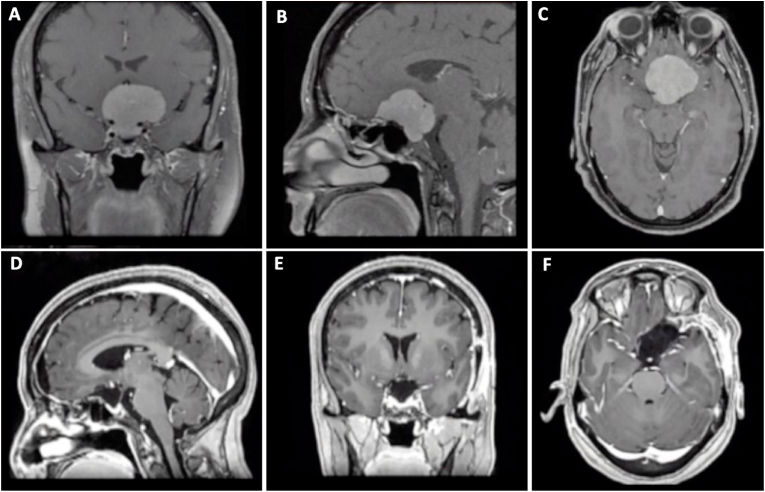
(A–C) Preoperative MRI: from left to right: coronal, sagittal, and axial views from a T1WI C+ showed an extra-axial lesion on the tuberculum sellae region, with suprasellar extension which obliterates the floor of the third ventricle, and an asymmetric growth over the dorsal portion of the left ICA. Encasement of both ICA can be observed. (D–F) Postoperative MRI: from left to right: axial, coronal, and axial views from a T1WI C+ in which gross total resection is observed.
